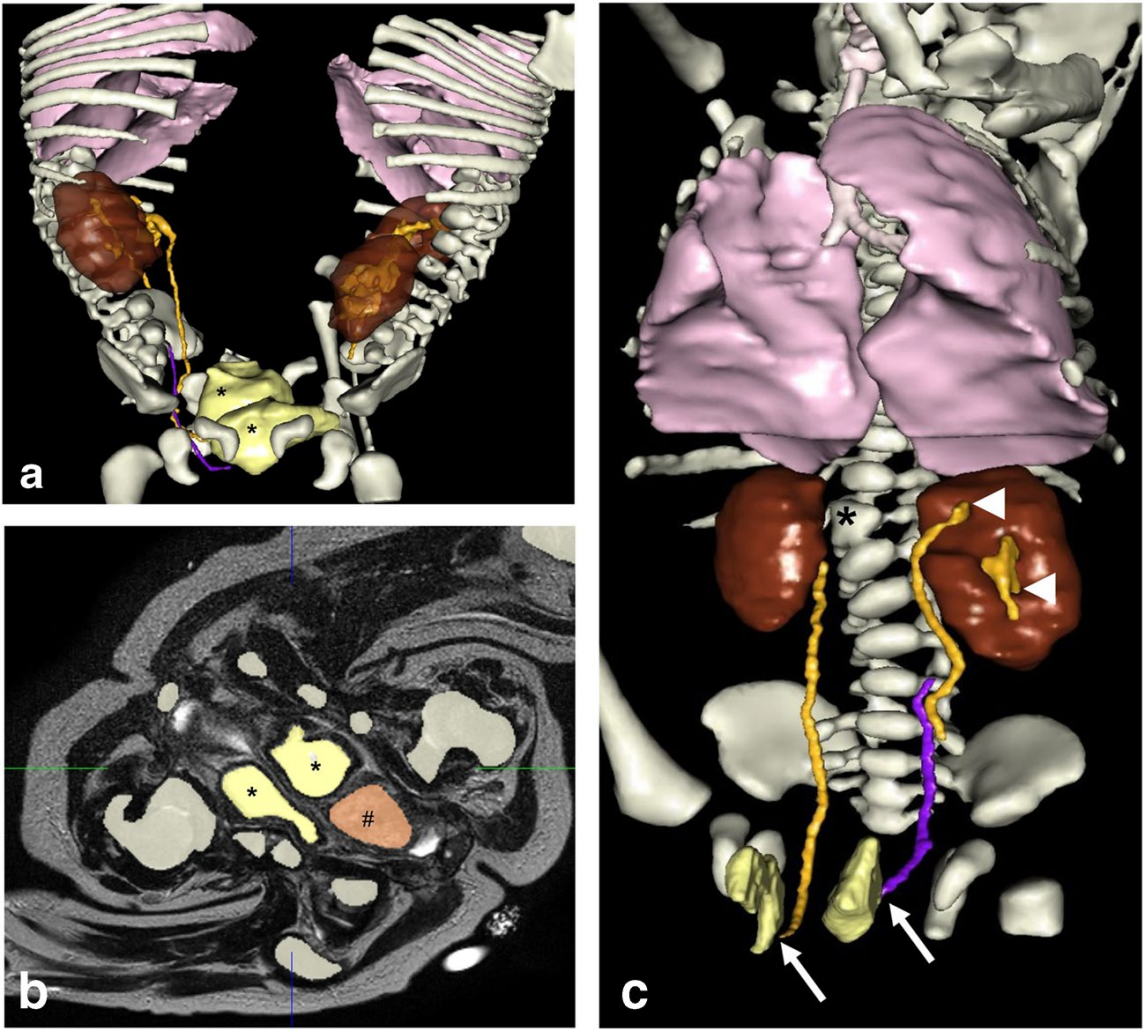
Imaging of 4-month-old ischiopagus conjoined twin boys (same patients as in Fig. 7). a Color-coded 3-dimensional (D) image extracted from a delayed phase computed tomography (CT) study. Each baby has two kidneys. There are two urinary bladders (*asterisks*) seen in the common pelvis. b Axial high resolution-T2-weighted magnetic resonance image with color overlay shows the anatomy of the conjoined pelvis. There are two urinary bladders (*asterisks*) and a single rectum (*#*). c Color-coded 3-D CT image of the left twin reveals each ureter draining into a separate bladder (*arrows*). The left kidney has a duplex collecting system (*arrowheads*) and proximal ureteric duplication. Note the presence of a hemivertebra (*asterisk*) (Visible Patient®, Strasbourg, France. *Ivory* bony skeleton, *brown* kidneys, *pink* lungs, *tan* rectum, *orange* ureters, *violet* ureter, *yellow* urinary bladders)
